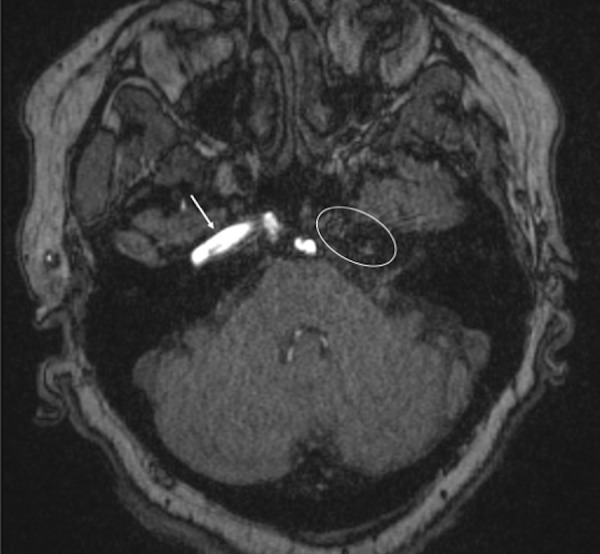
Axial 3D time of flight MRI of the head, noting absence of the carotid canal and internal carotid artery at the skull base (oval). For comparison, note the right-sided internal carotid artery (arrow) at the skull base.
